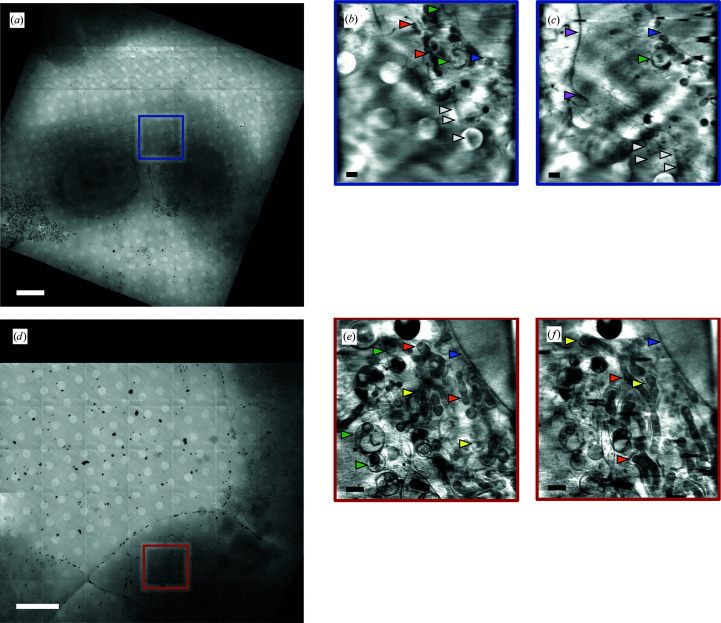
X-ray mosaics (7 × 7 frames) from areas of interest collected from two different grids bearing vitrified U2OS cells with (*a*) the 40 nm and (*d*) the 25 nm zone plate. Data were collected in the highlighted areas in (*a*) and (*d*) and representative slices from the reconstructed tomograms at different *z* heights can be seen in (*b*), (*c*), (*e*) and (*f*) (colour-coded to match the originating mosaic). The arrows used to highlight the cellular features are nuclear membrane in blue, outer cell membrane in purple, endoplasmic reticulum in yellow, mitochondria in orange, multivesicular bodies in green and actin bundles in white. Scale bars are 10 µm for (*a*) and (*d*) and 1 µm for (*b*), (*c*), (*e*) and (*f*).
